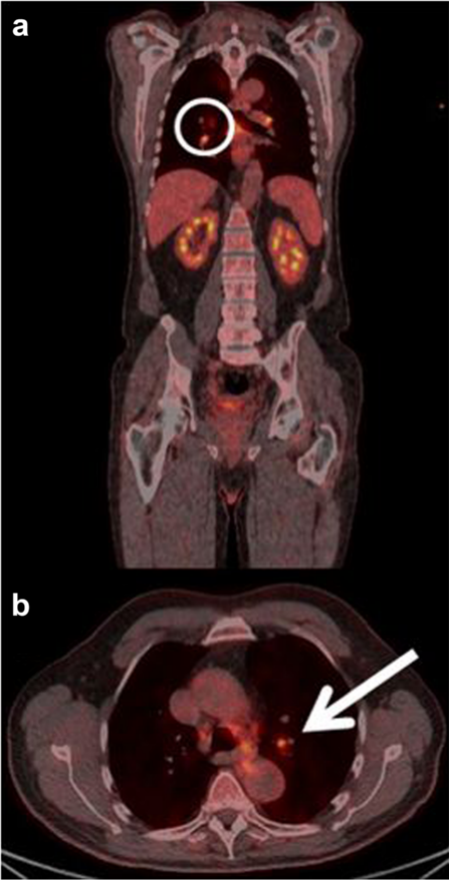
a Coronal-fused FDG-PET/CT showing the region of interest (*circle*). b Axial-fused FDG-PET/CT showing increased uptake in the hilar lymph nodes (*arrow*)
